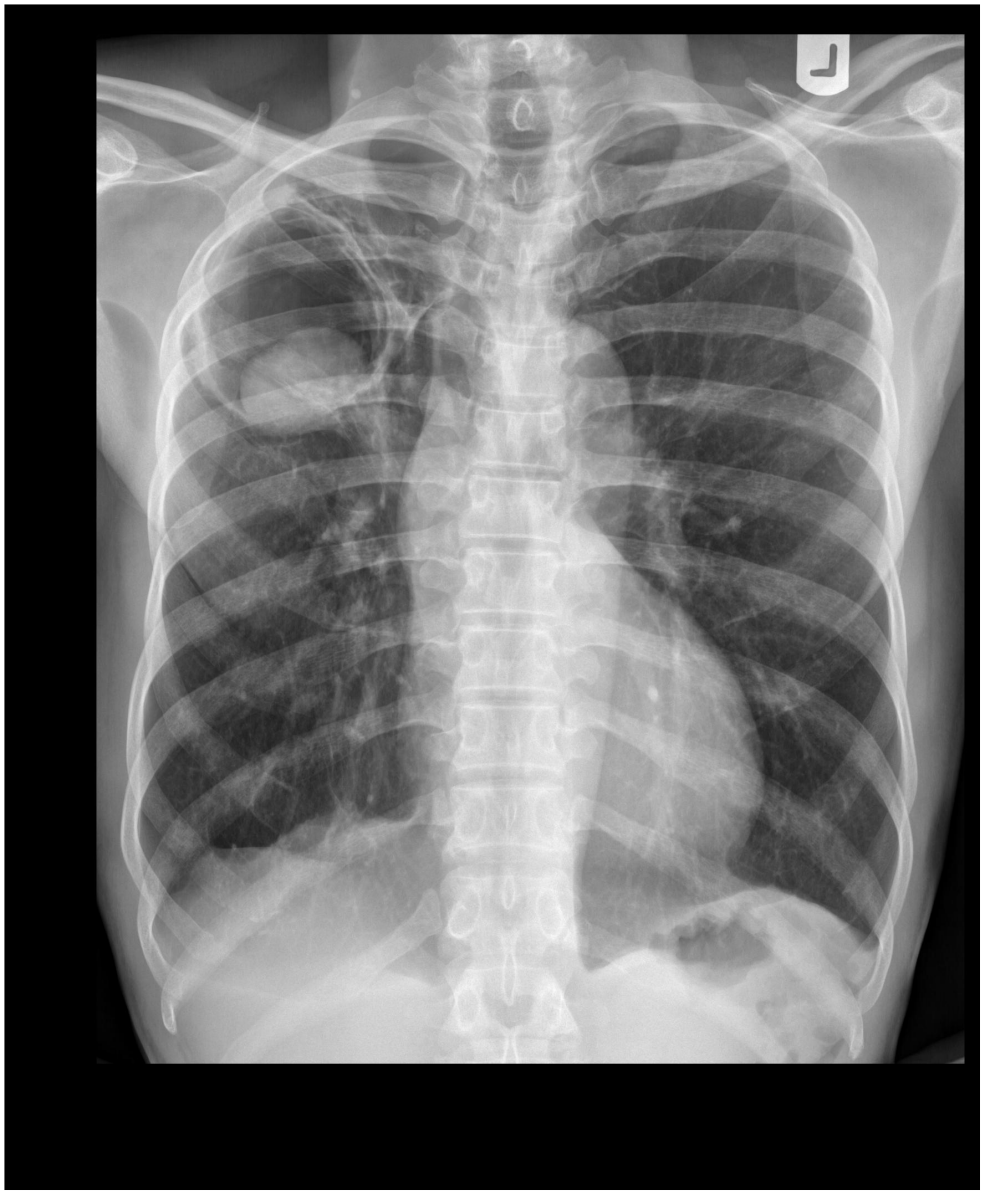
Chest radiography showing a fungus ball with an air crescent in the right upper lobe.
